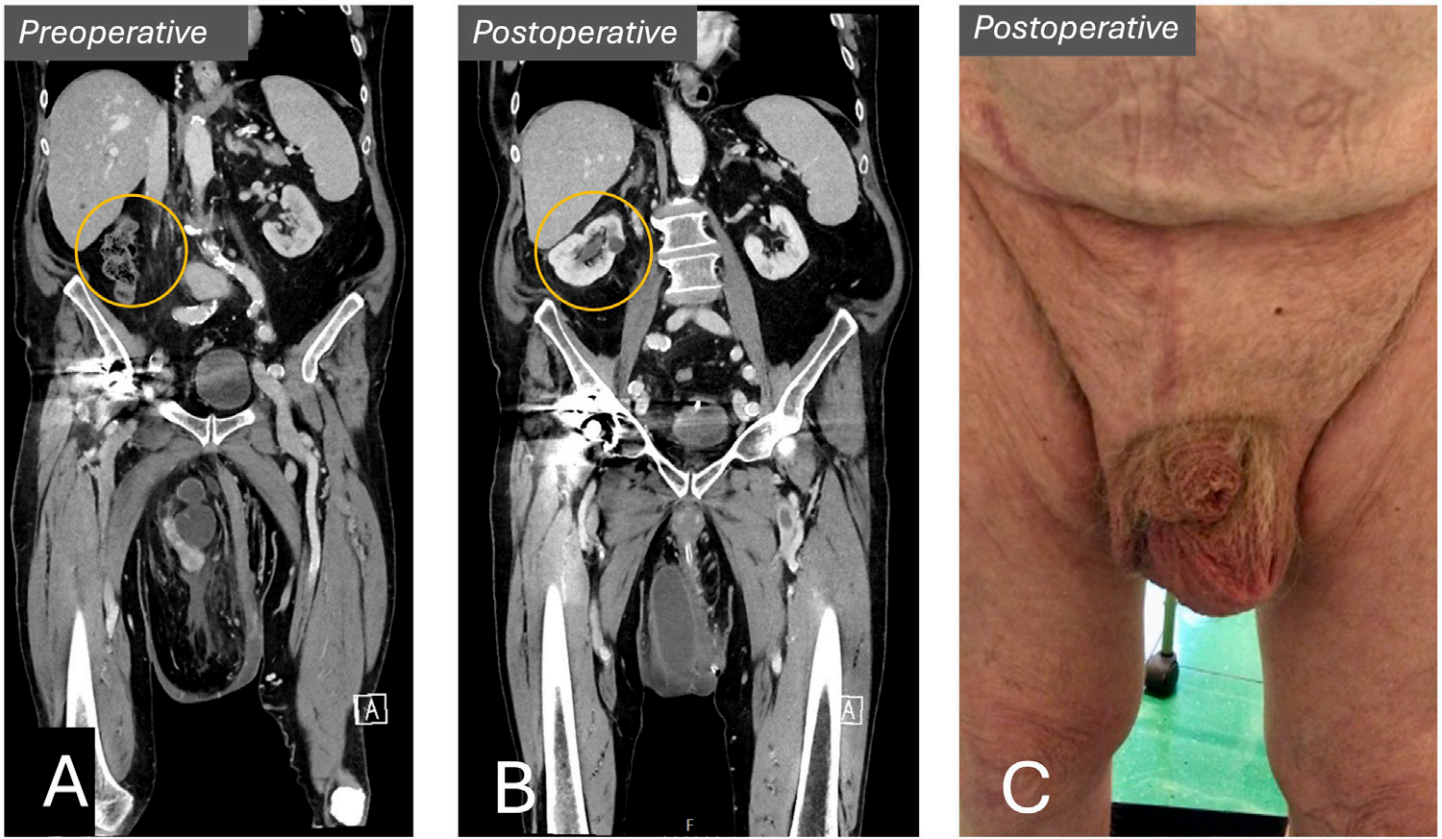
Preoperative vs. postoperative. (A) Preoperative CT-scan showing kidney within scrotum. (B) Postoperative CT-scan showing right kidney in its normal anatomical position within the right flank. (C) Postoperative result.
